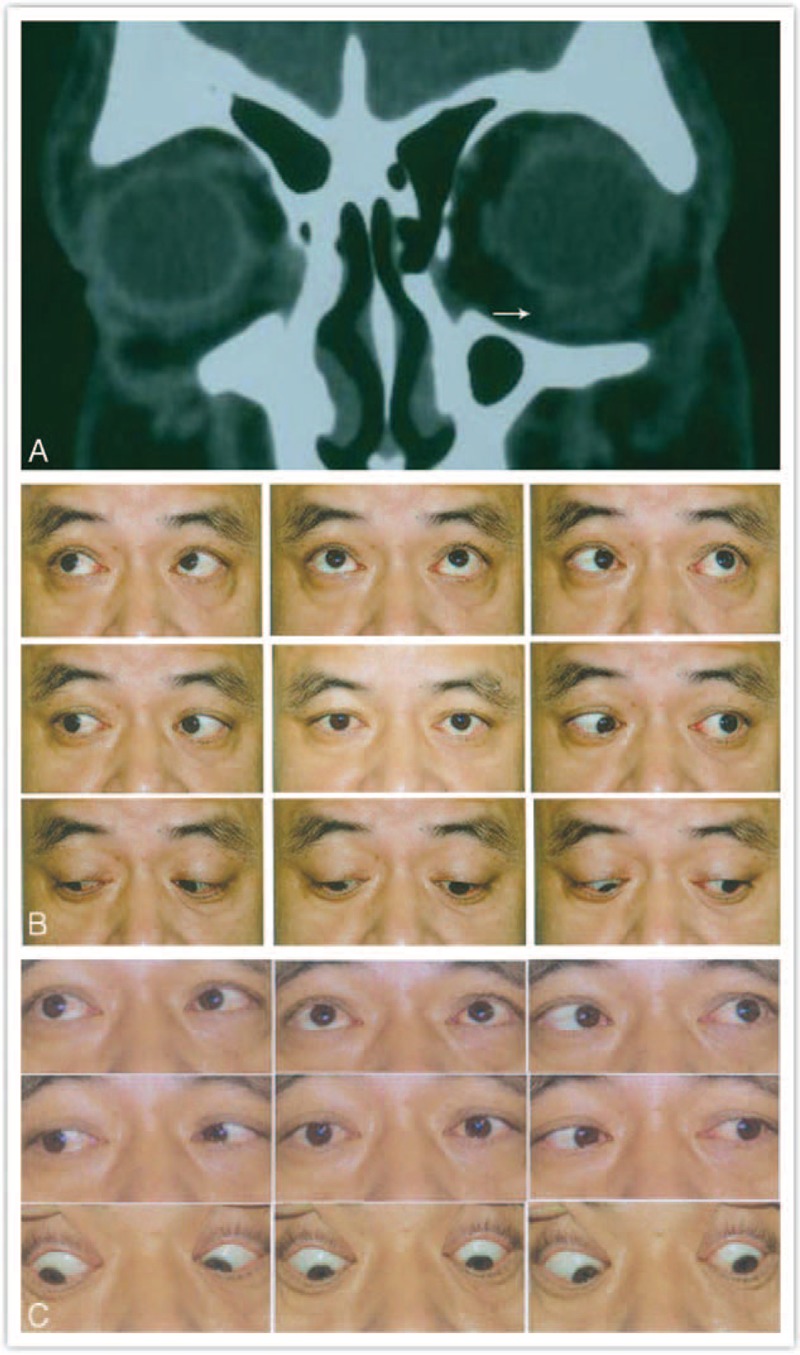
A–C: Preoperative and postoperative alignment and CT of case 11 with a total rupture of the left inferior rectus muscle. A: Orbital CT showing an impinged left inferior rectus muscle. B: Before surgery, 18 PD left hypertropia and a scale of −3 motility defect of left inferior rectus muscle, together with left lower eyelid retraction. C: 15 months after surgical repair (end-to-end muscle anastomosis and a 4 mm resection of the ruptured inferior rectus muscle), orthotropia, and normal ocular motility. CT = computerized tomography, PD = prism diopter.
